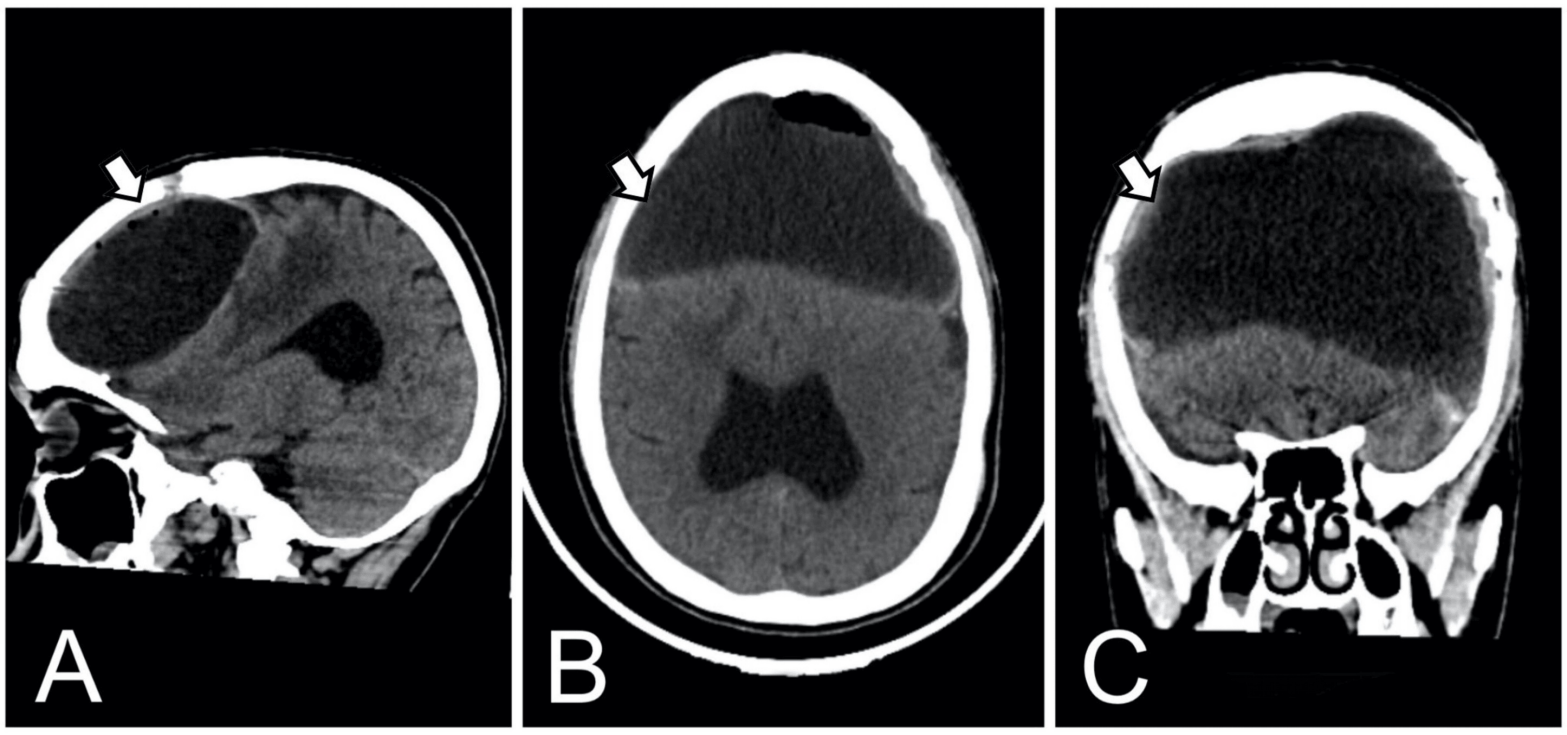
Postoperative Non-contrast Cranial Computed Tomography (CT) CT scans with sagittal (A), axial (B), and coronal (C) views. The images revealed the presence of arachnoid cysts occupying the frontal region, without evidence of rebleeding.
